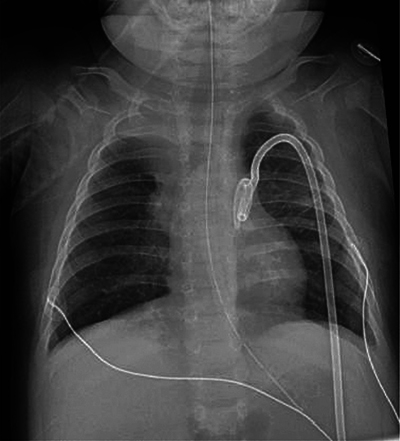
Chest radiograph. Total recovery of the free air throughout the body due to drainage and tissue resorption.
